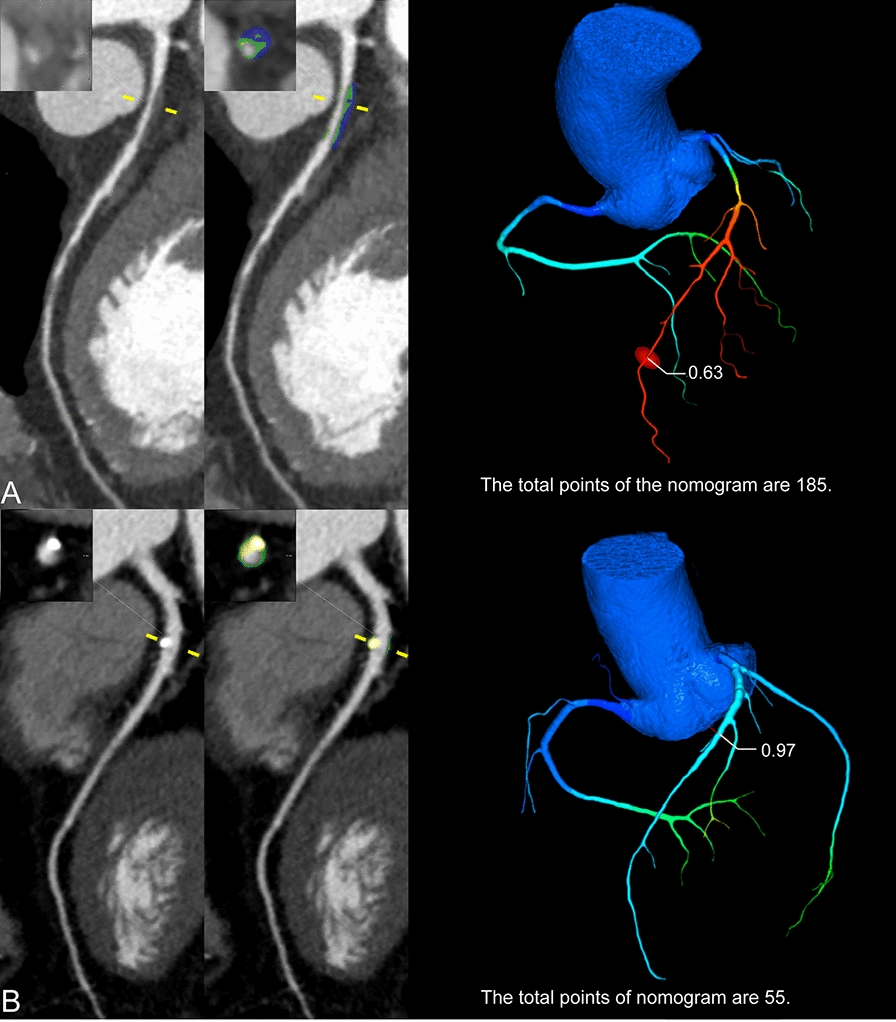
Representative cases of diabetic patients with and without MACE. (A) CCTA of a 63-year-old male with stable angina showed multiple obstructive stenosis at proximal and middle LAD. Plaque characterization revealed the presence of LAP for the proximal lesion (blue content) and the distal CT-FFR value was 0.63. The total points of the proposed nomogram were 185. This patient underwent late revascularization of proximal LAD lesion 2.29 years later due to aggravated angina symptom. (B) CCTA of a 79-year-old male with stable angina showed mild stenosis at proximal LAD. Plaque characterization revealed the absence of any HRP feature and the distal CT-FFR value was 0.97. The total points of the proposed nomogram were 55. The patient did not develop MACE at a follow-up of 2.72 years. CCTA = coronary computed tomography angiography; CT-FFR = computed tomography fractional flow reserve; HRP = high-risk plaque; LAP = low-attenuation plaque; LAD = left anterior descending; MACE = major adverse cardiac events
